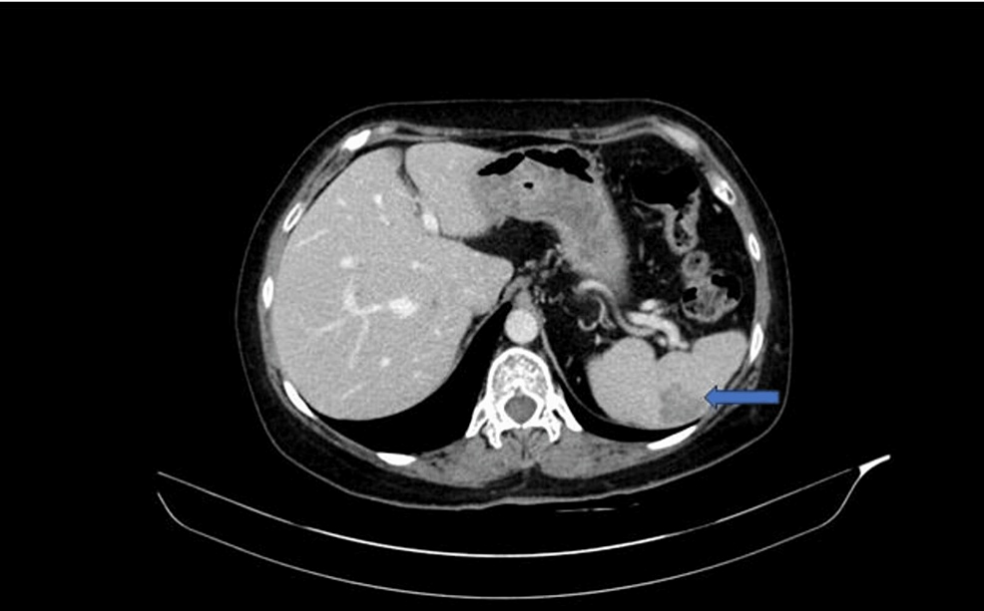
CT abdomen/pelvis with contrast indicates a hypodense mass lesion in the spleen (arrow).
